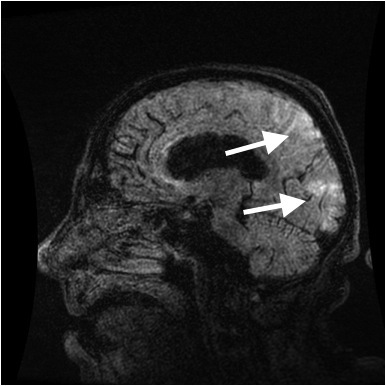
T2 weighted magnetic resonance imaging of Patient 2, with hyperintensity of posterior parietal and occipital lobes.
